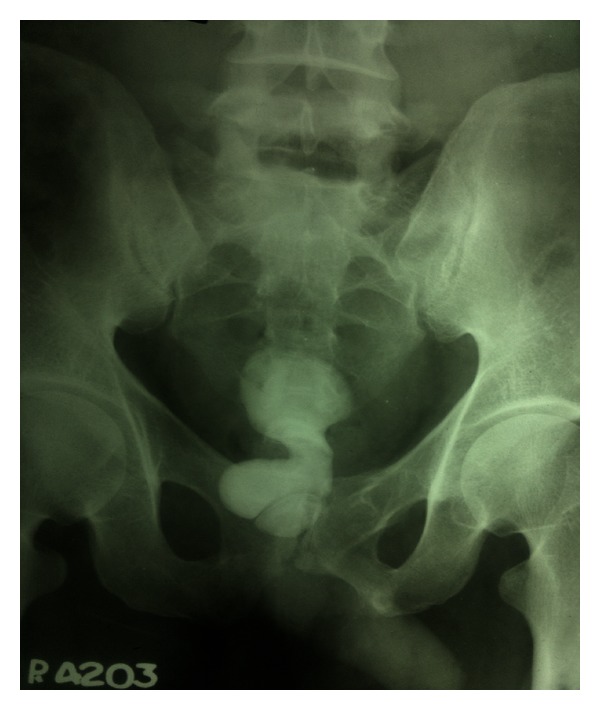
Erect X-ray KUB showing a giant calculus in the lower urinary tract.
